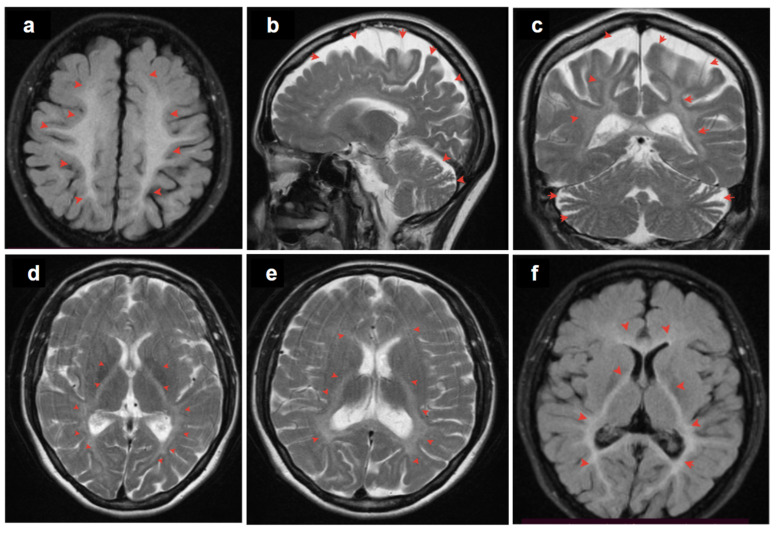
MRI images of the brain: diffuse, bilateral hyperintensities in the subcortical white matter (a); cortical and cerebellar atrophy (b,c); coronal view of the posterior subcortical and periventricular T2 hyperintensities (c); posterior limb of the internal capsule and periventricular areas (d–f). Red arrows point to the affected regions.
